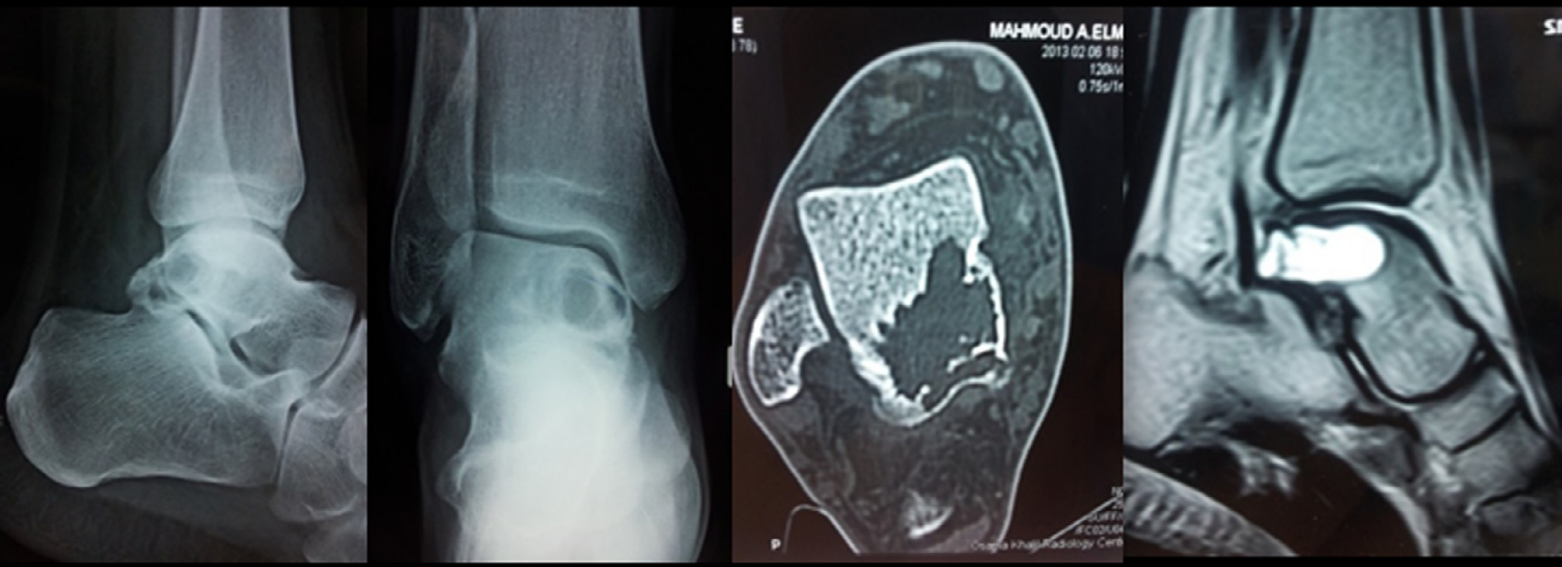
A male patient 28 years old. Plain radiographs, CT scan, and MRI revealed a large aneurysmal bone cyst involving the posterior third of talus.
